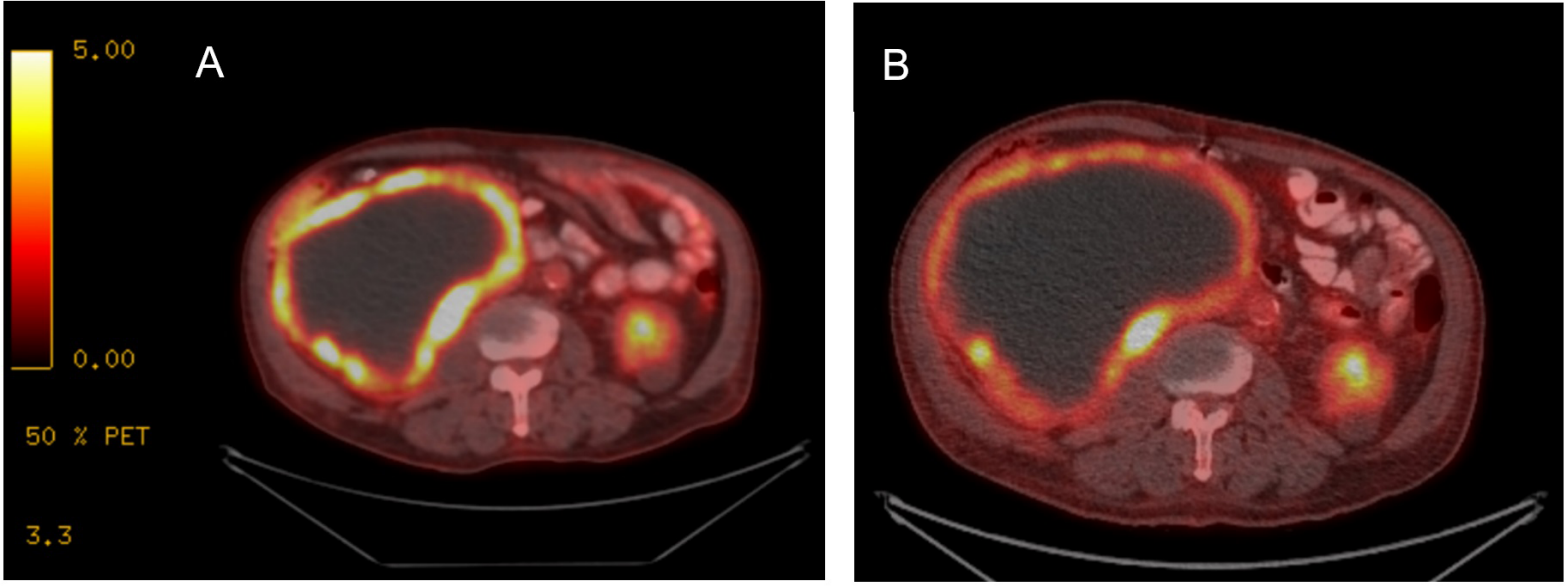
FDG PET/CT with treatment response to imatinib. (A) At diagnosis, FDG PET/CT imaging demonstrated a large right renal tumor with peripheral enhancement and necrosis. (B) After treatment initiation, while tumor size is comparably similar, peripheral avidity has decreased, consistent with overall treatment response.
